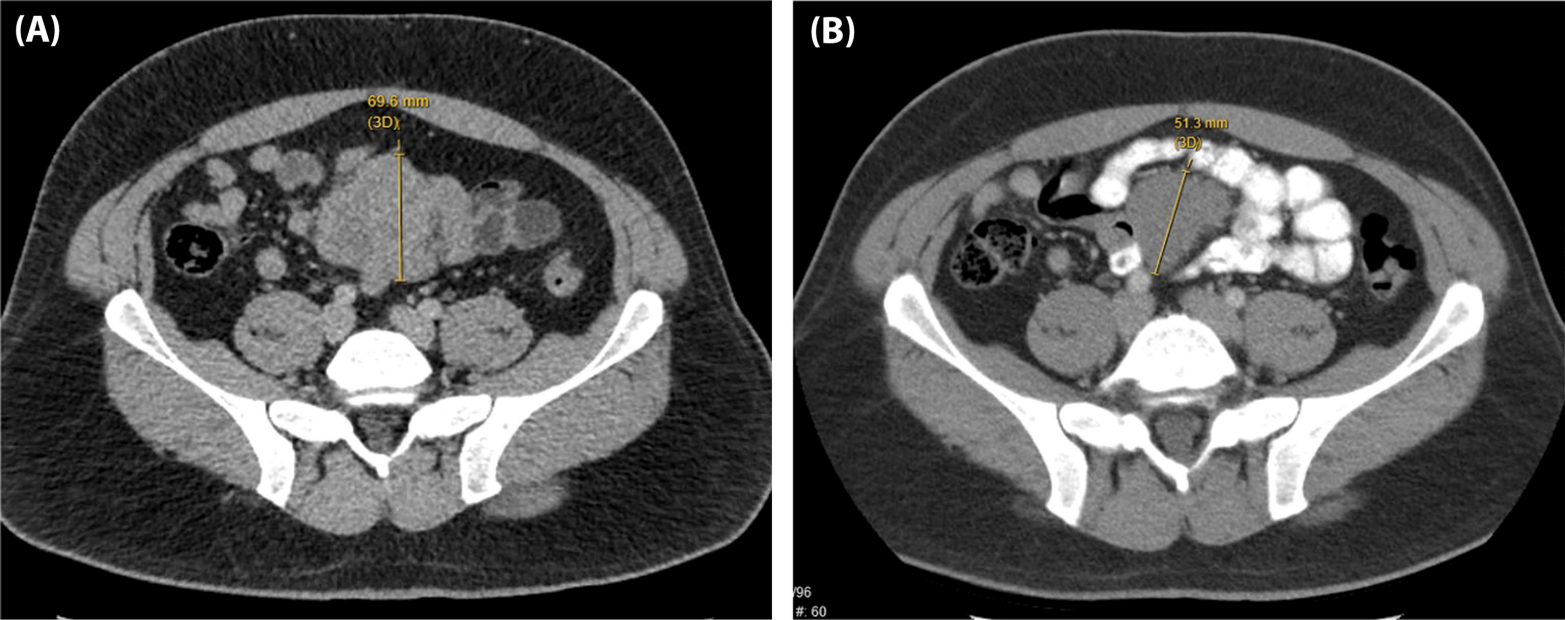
A 37-year-old man with BRAF-wildtype, metastatic melanoma with lung, bone, mesentery, and brain involvement who received dose 1 of ipilimumab/nivolumab on 06/23/17 and dose 2 on 07/15/17. (A) Baseline CT abdomen/pelvis on 05/23/17 and (B) CT abdomen/pelvis on 07/11/17.
